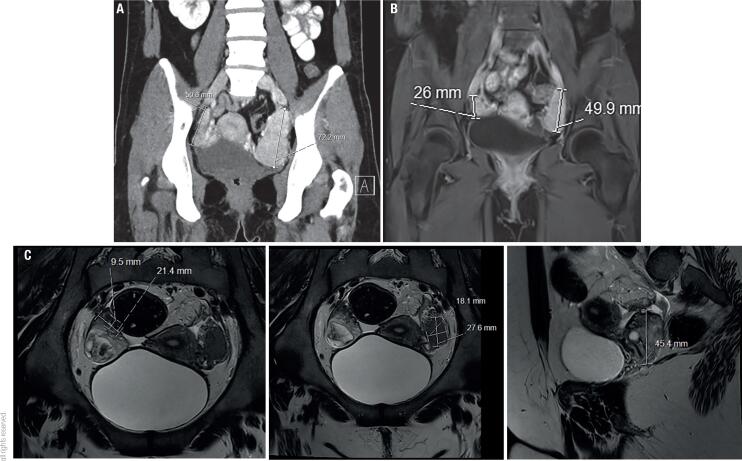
(A) CT scan at admission showing bilateral para-ovarian masses) 62 x 36 x 72 mm on the left, 56 x 22 x 32 mm on the right). (B) MRI scans 3 months post-admission showing as isointense in mass T1 and hypointense in T2, with early enhancement and rapid washout and significant shrinkage of the masses. (C) MRI scan 1-year post-admission showing shrinkage of the masses.
